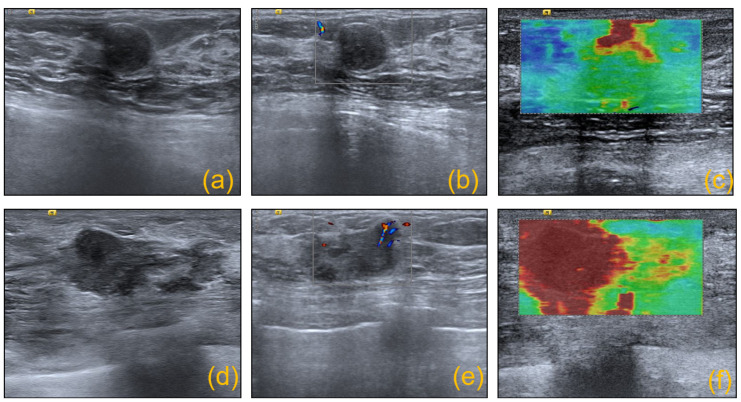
Multimodal ultrasound examples of benign (top row) and malignant (bottom row) breast lesions. (a,d) BUS; (b,e) CDFI; (c,f) UE.
